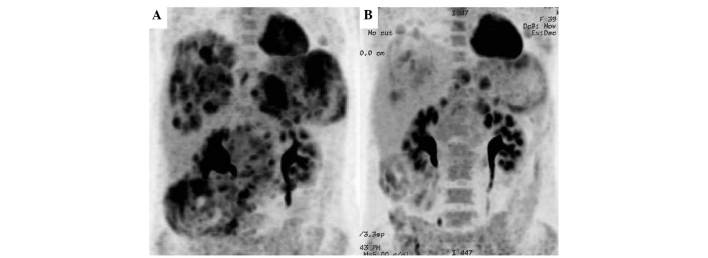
FDG-PET analysis. (A) Extremely high FDG uptake by the tumors was detected on FDG-PET analysis. (B) Following chemotherapy and transarterial embolization, a reduction of FDG uptake was observed. FDG-PET, 18-fluorodeoxyglucose-positron emission tomography.
